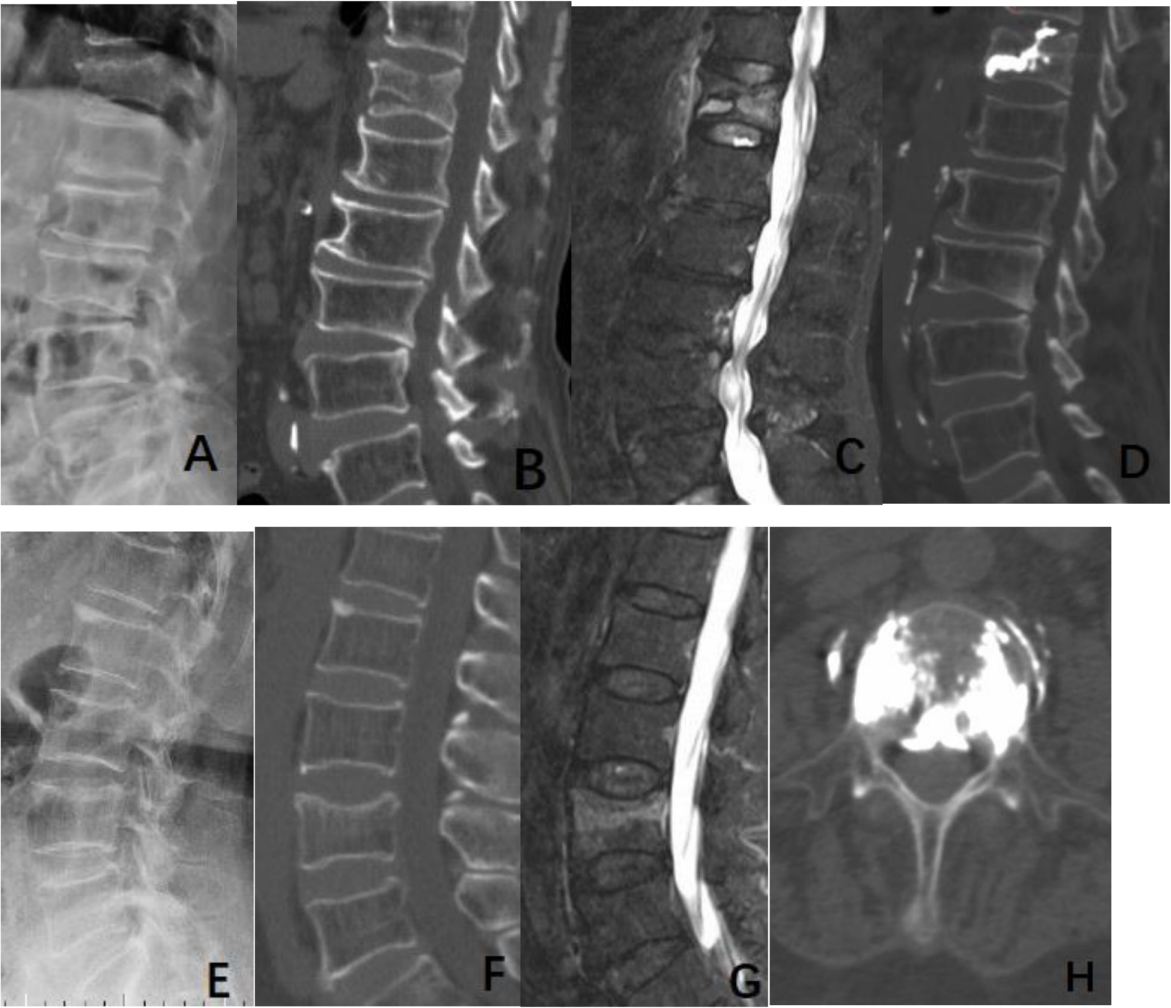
Case 1 (a, b, c, d) was an 88-year-old male patient. A moderate and biconcave type fracture in T12 was shown on lateral radiograph (a). An IVC in T12 was confirmed on sagittal CT reconstruction (b) and MRI (c). An area similar to fluid in this IVC was demonstrated on sagittal MRI (c). Leakage was detected through the upper endplate of T12 vertebra on postoperative CT (d). Case 2 (e, f, g, h) was a 71-year-old female patient. There was evidence of a mild and wedge type fracture in L4 on lateral radiograph (e). A L4 fracture with absence of the IVC was confirmed on sagittal CT reconstruction (f) and MRI (g). Leakage was detected into spinal canal and segmental vein on postoperative CT (h)
